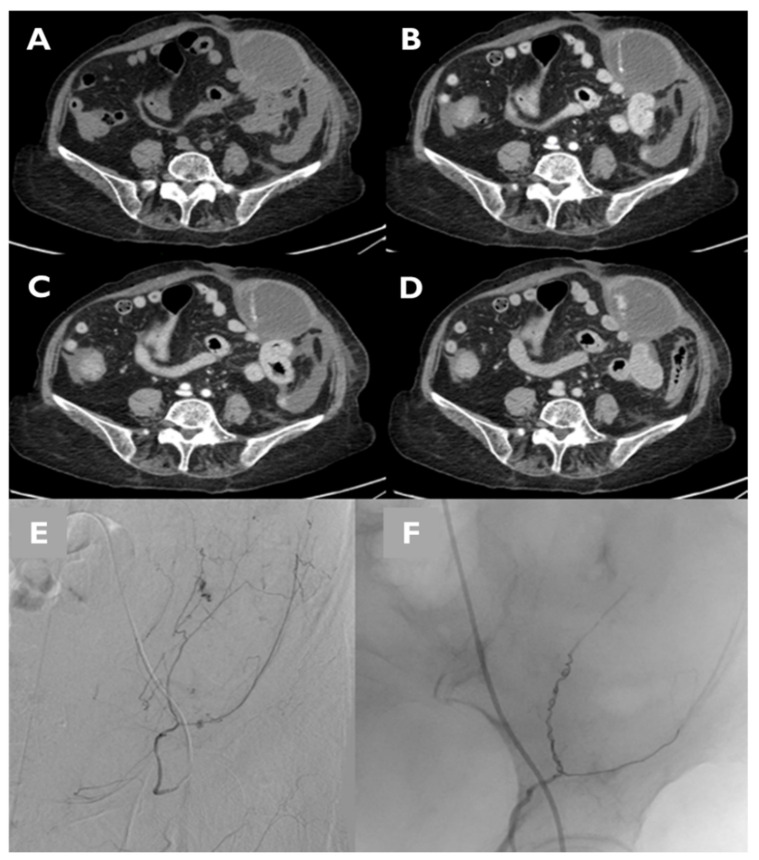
CT and DSA images of a patient presenting with hypovolemic shock. Evidence of a large hematoma within rectus abdominis below the arcuate line in axial CT images. The pre-contrast phase shows the extension and location of the hematoma (A). The contrast-enhanced acquisition at the arterial (B), portal (C) and delayed phase (D) allow the active bleeding to be detected. Angiography of the same patient shows contrast blush from branches of the inferior epigastric artery (E). TAE was performed using 3-mm coils and a Gelatin sponge (F).
